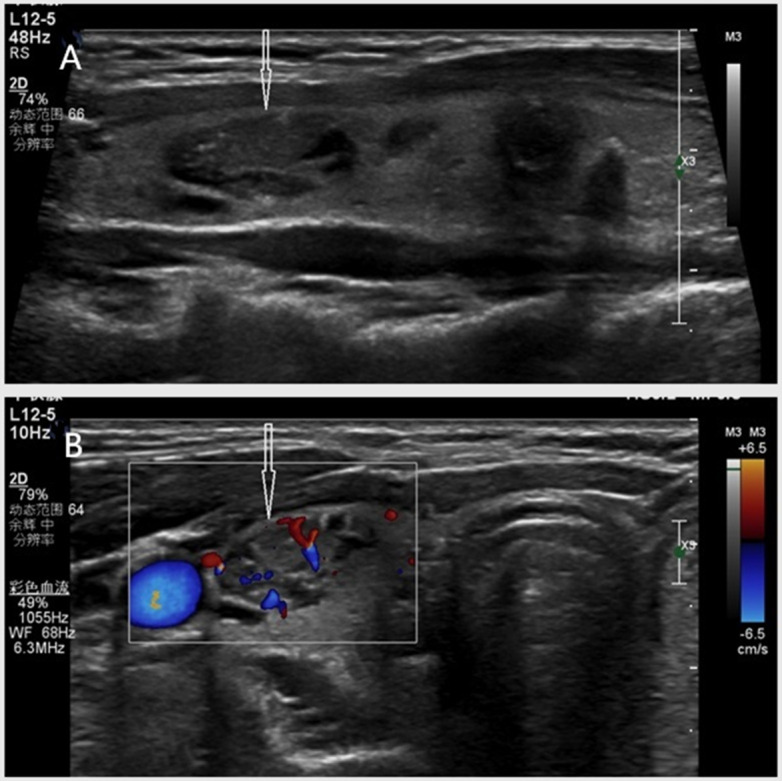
(A–B) A 45-year-old female with a pathologically confirmed thyroid nodule (follicular hyperplasia). Ultrasound demonstrates a hypoechoic nodule (arrows indicate) in the right thyroid lobe with the following features: elliptical shape (no taller-than-wide), mixed solid-cystic composition, scattered punctate hyperechoic foci, well-defined margins, and dimensions of 16.8 × 9.9 × 8.2 mm. No posterior acoustic features observed. Classification: classical C-TIRADS 4a, modified C-TIRADS 4a, K-TIRADS 4.
